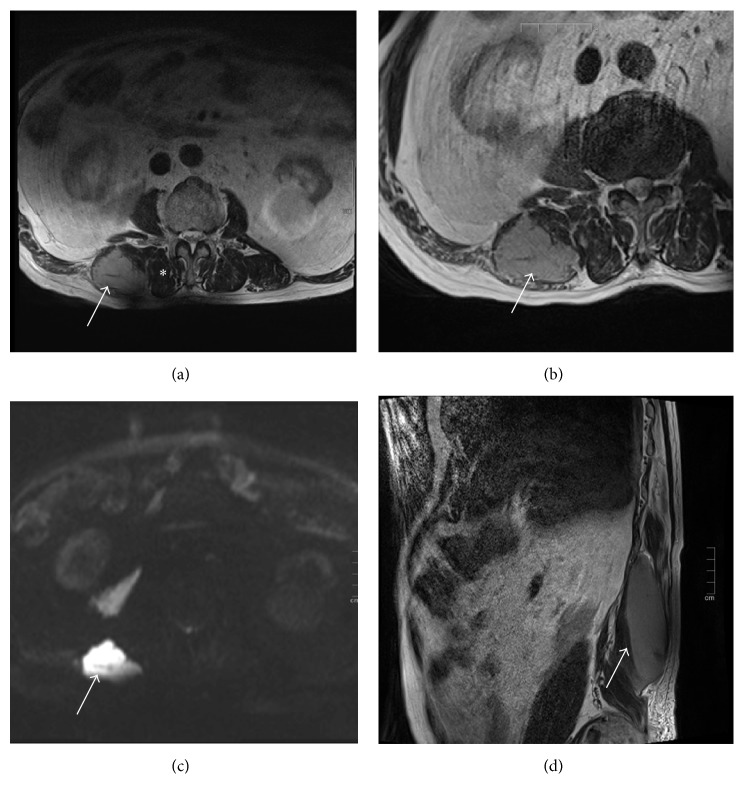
Plain magnetic resonance images of the abdomen on admission. (a) Plain axial T1-weighted image of the abdomen revealed a mass in the right psoas muscle. The mass exhibited higher intensity (white arrow) than the adjacent normal musculature (asterisk). (b) The mass also showed mildly higher intensity on T2-weighted imaging than the adjacent normal musculature (white arrow). (c) The mass showed markedly high intensity on diffusion-weighted imaging (white arrow). (d) Plain sagittal T2-weighted image of the abdomen revealed an oval-shaped mass with homogenous, mildly high intensity inside the right psoas major muscle (white arrow).
